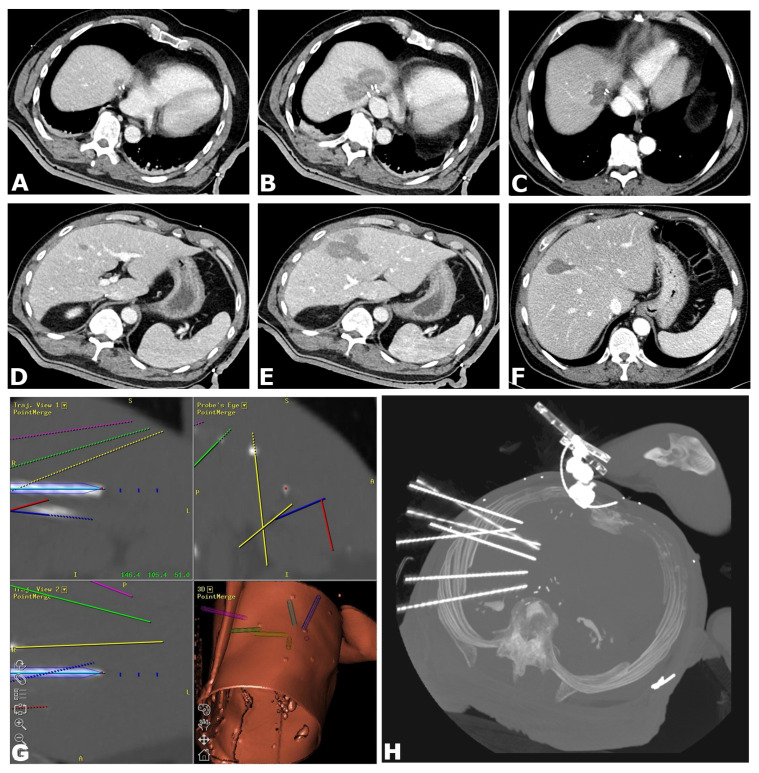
A 57-year old male with three colorectal cancer liver metastases (max 1.8 cm). (A) CT-image of the contrast-enhanced planning CT scan with a 1.8 cm recurrent metastasis following hepatic resection close to the central hepatic veins. (B) Immediate post-ablation and (C) 12-month contrast-enhanced CT scans showing no evidence of residual or recurrent disease. (D) Contrast-enhanced planning CT image showing a further 1.5 cm metastasis in segment V. (E) Immediate post-ablation and (F) 12-month CT scan showing no evidence of residual or recurrent disease. (G) Images from the navigation system depicting fused CT images of the needle control and planning scans with an example of a longitudinal planned trajectory (light blue) and the corresponding probe`s eye view (red dot) with perfect alignment. (H) maximum intensity projection image of the non-enhanced control CT scan showing all seven coaxial needles in position.
